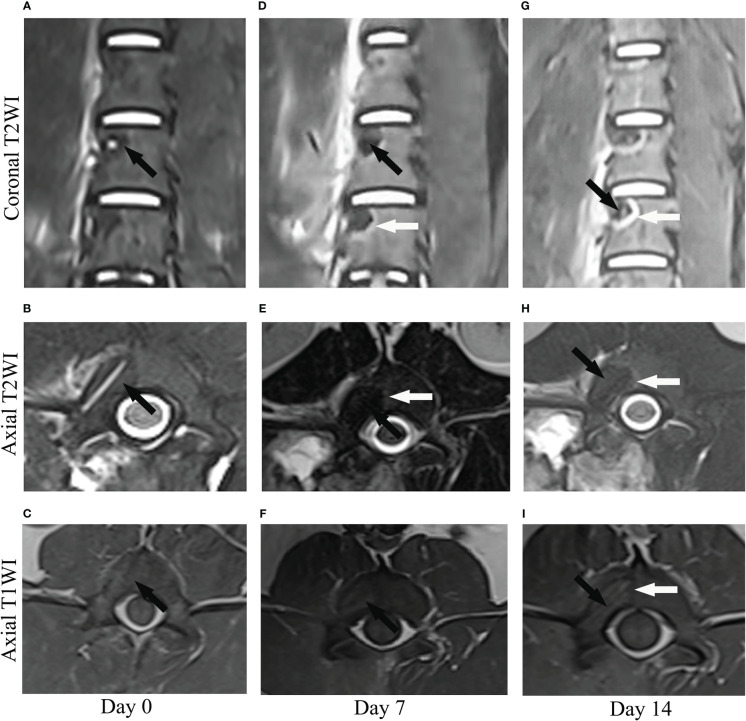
The image manifestation of a lesion on coronal and axial T2-weighted images (T2WI) and axial T1-weighted images (T1WI) at different time points (day 0, 7, 14) after radiofrequency ablation. (A–C) On day 0, the lesion showed hypointensity (black arrow) on coronal and axial T2WI and isointensity (black arrow) on axial T1WI. (D–F) On day 7, the lesion demonstrated hypointensity (Black arrow) at the center with hyperintensity (white arrow) at the periphery on coronal and axial T2WI and heterogenous intensity at the center with slightly hypointensity at the periphery on axial T1WI (Black arrow). (G–I) On day 14, the peripheral ring (white arrow) which manifested as hyperintensity on coronal and axial T2WI and hypointensity at the center was clearer than that of day 7.
